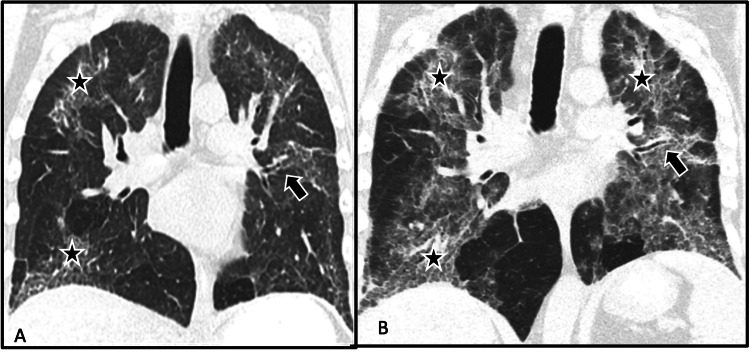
Progression of disease. (A) Coronal CT image at baseline showing few early ground glass reticular densities in the periphery predominantly at the lung apices and lung bases (black star) with mild traction bronchiectasis (black arrow). (B) Coronal CT image two years later when the patient presented with worsening dyspnea. The image shows the progression of lung disease with progression in the distribution of ground glass and reticular densities (black star) now involving the central regions in the upper and mid lung bilaterally with signs of fibrosis and mild traction bronchiectasis (black arrow).
